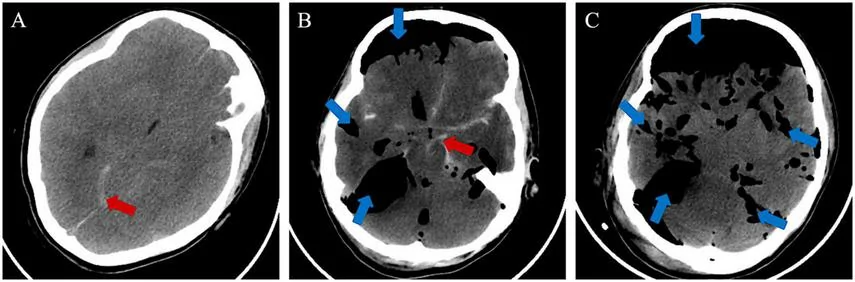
Progression of extensive pneumocephalus on serial cranial CT scans. (A) Day 1 (admission): Subarachnoid hemorrhage is noted (red arrow), and no evidence of pneumocephalus or skull fracture is observed. (B) Day 19: Significant intracranial gas accumulation is visible, distributed in the frontal, temporal, and occipital lobes (blue arrow); subarachnoid hemorrhage is also noted (red arrow). (C) Day 32: Marked progression of pneumocephalus is evident, with extensive gas accumulation throughout the intracranial cavity (blue arrow).
